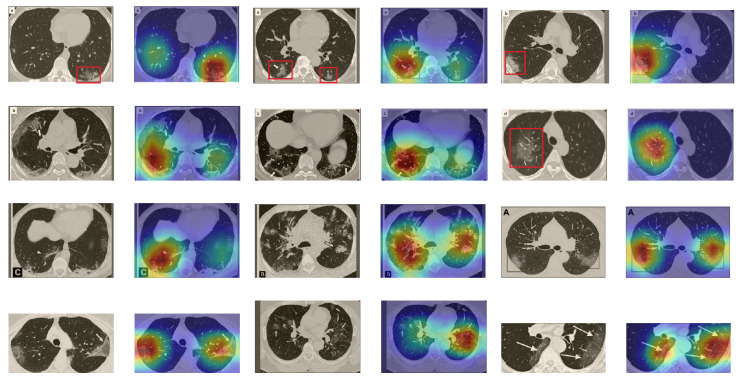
Examples of CT images taken from these two publications [61,62]. The CT images were correctly classified as COVID-19 cases, and the abnormal regions are accurately detected as in the localization maps.
